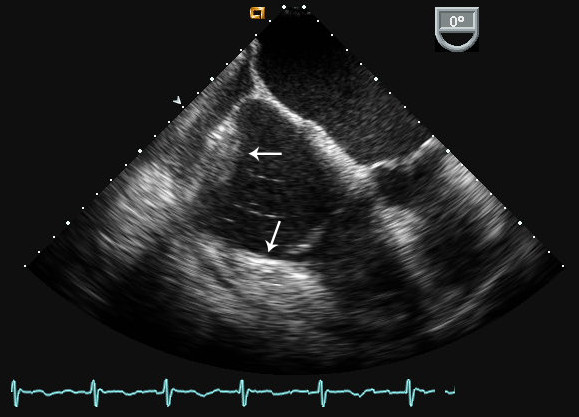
Transesophageal echocardiogram (midesophageal view) with echodensities in the right atrial free wall, right ventricular free wall and atrioventricular groove (arrows).
